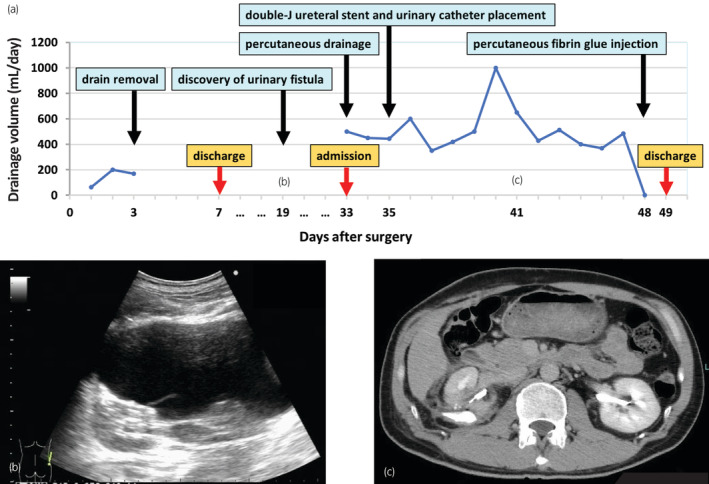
Clinical course of the case. (a) Time‐course change in therapies and drainage volume, (b) Right kidney ultrasound image on the 19th postoperative day. A hypoechoic image suggestive of urinary fistula is observed around the kidney, (c) Computed tomography on the 41th postoperative day. Leakage of contrast medium is observed around the right kidney (coronal section, excretory phase).
